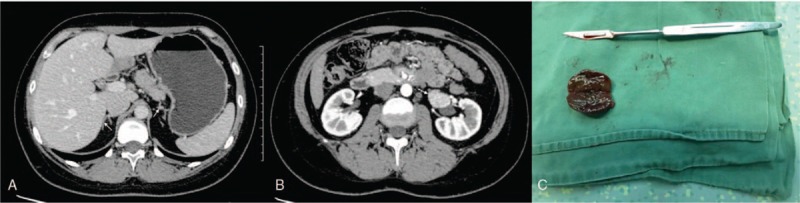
A: Abdominal CT of the patient. Enhanced CT showed a well-circumscribed round mass with a maximum diameter of 3.0 cm in the left renal hilum. B: CT scan revealed bilateral adrenal glands were atrophic. 1C: the ectopic adrenal tumor was resected during the operation, and was sized 3.0cm × 2.5cm × 1.5 cm. CT = computed tomography.
